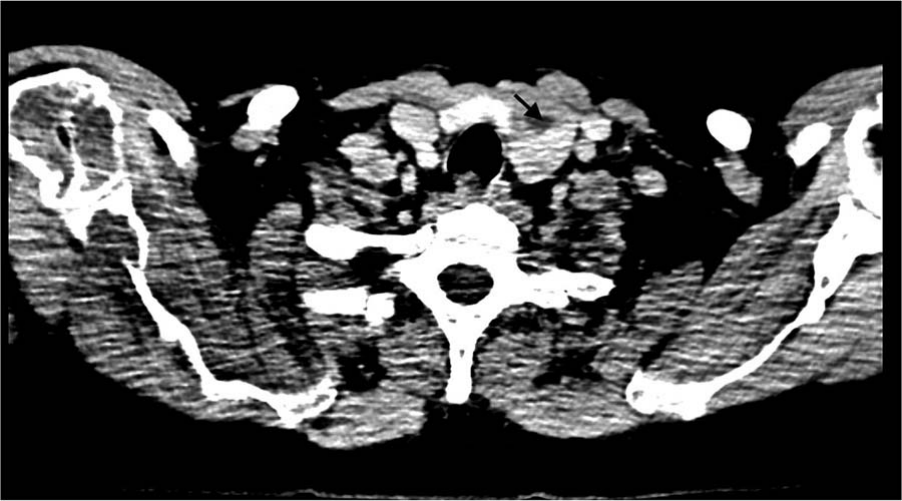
Neck CT (post-contrast, axial) localizing enlarged parathyroid glands. A 2.45 × 2.42 × 3.3 cm heterogeneously enhancing mass with central necrosis is seen in the postero-inferior aspect of the left thyroid lobe. The mass abuts the ipsilateral thyroid lobe and trachea without evidence of invasion. Carotid vessels and the esophagus are intact.
